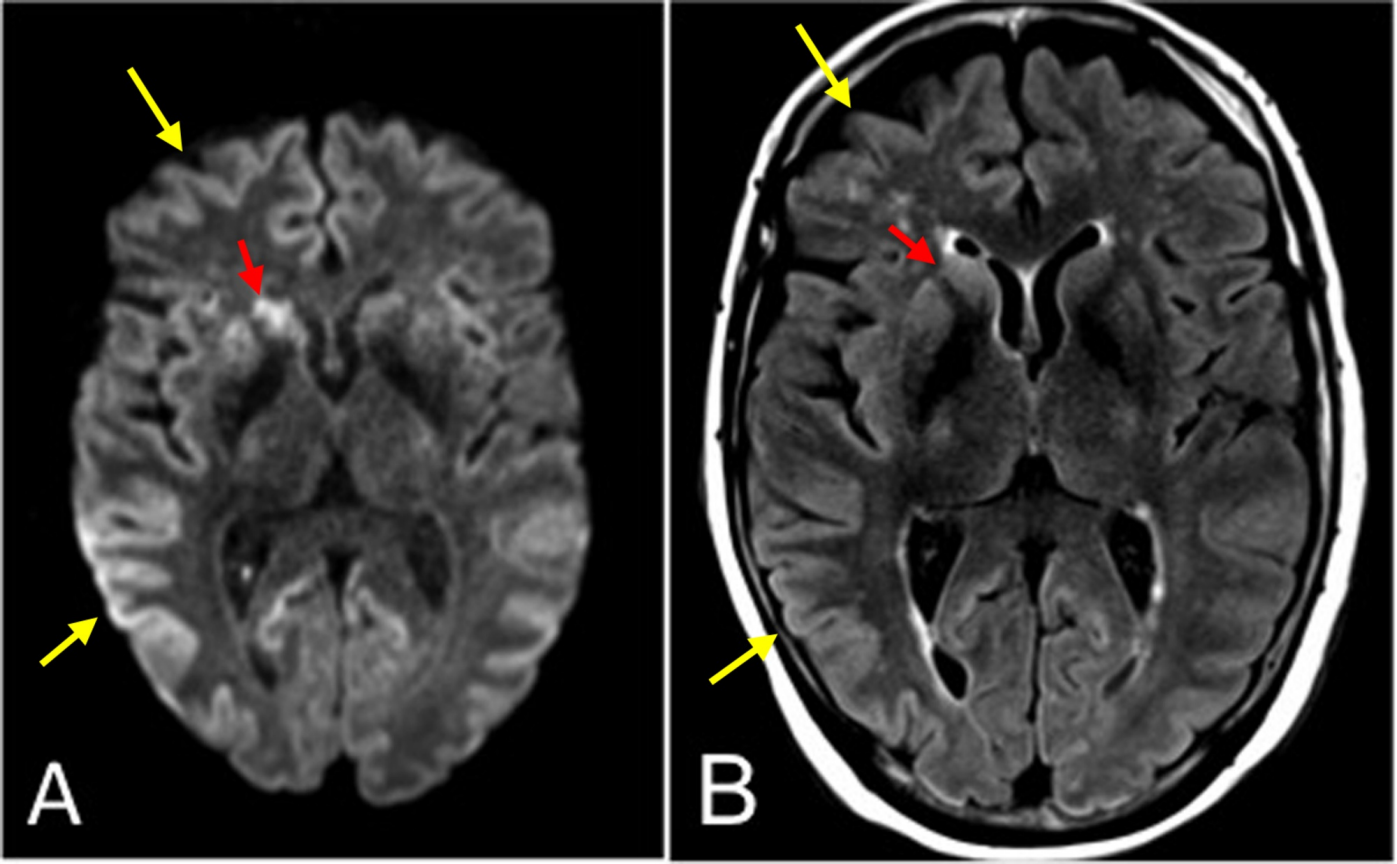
Axial magnetic resonance imaging (MRI) of Case 1. (A) Diffusion-weighted image (DWI) at the level of the basal ganglia demonstrating asymmetrically increased signal in the entire neocortex (yellow arrows) and striatum (red arrows). (B) T2-weighted fluid attenuation inversion recovery (T2-FLAIR) image with less corresponding hyperintense signal.
